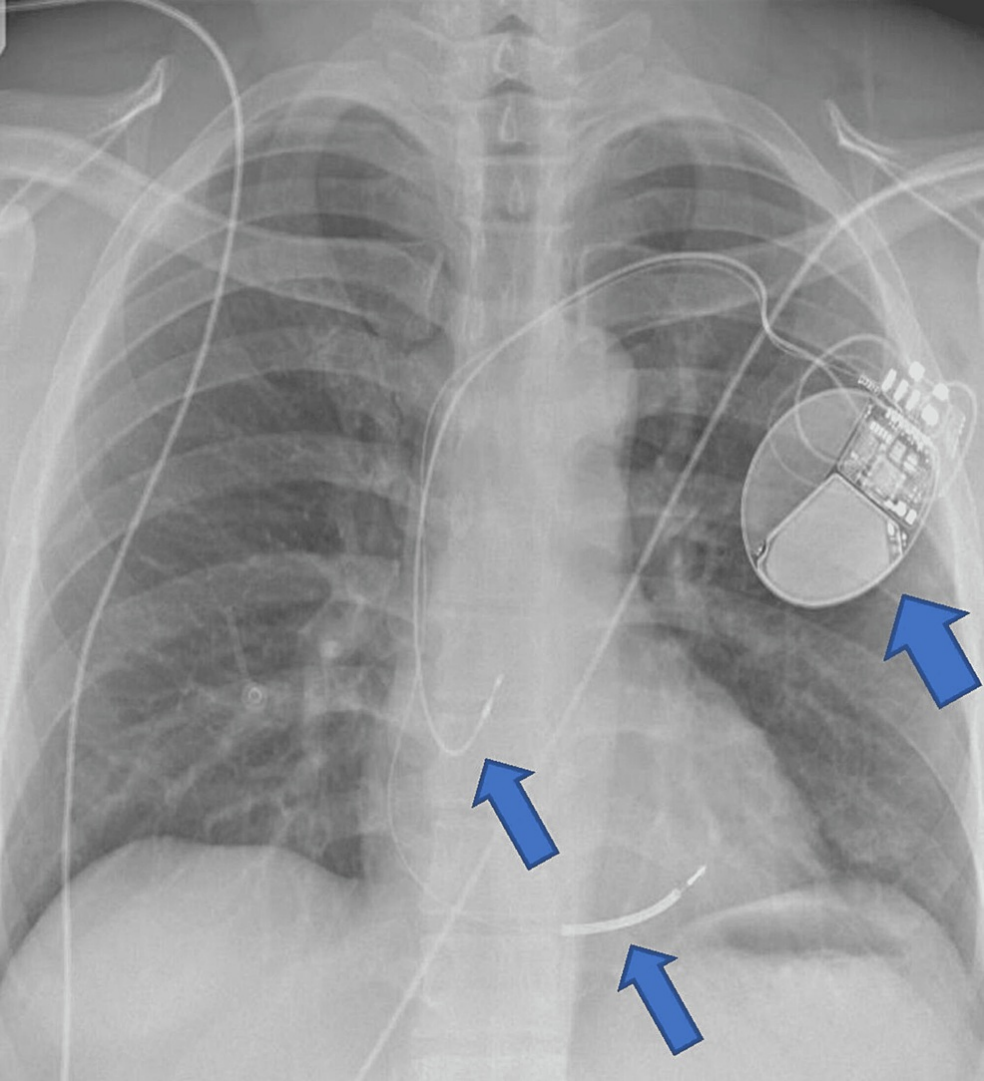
Post-ICD implantation chest radiography ICD: implantable cardioverter defibrillator
